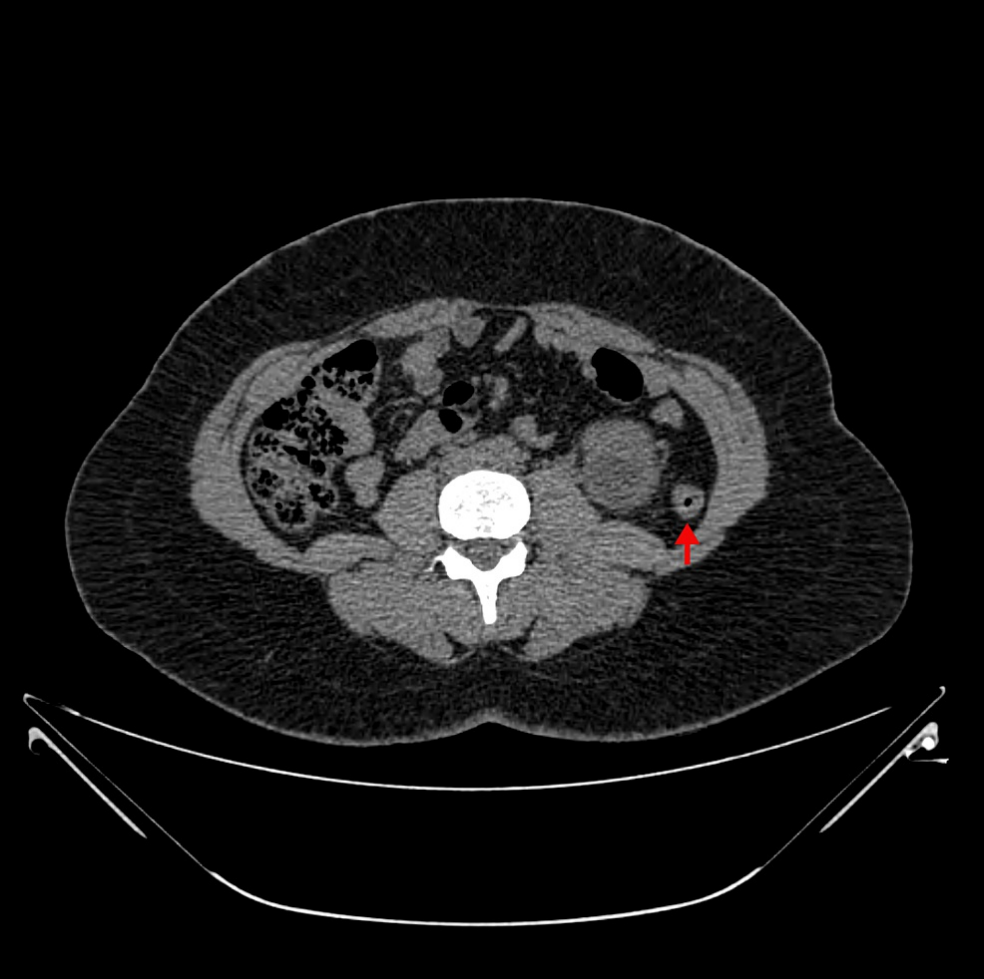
CT scan of abdomen - axial slice at lower pole showing left retro-renal colon with air in the lumen
